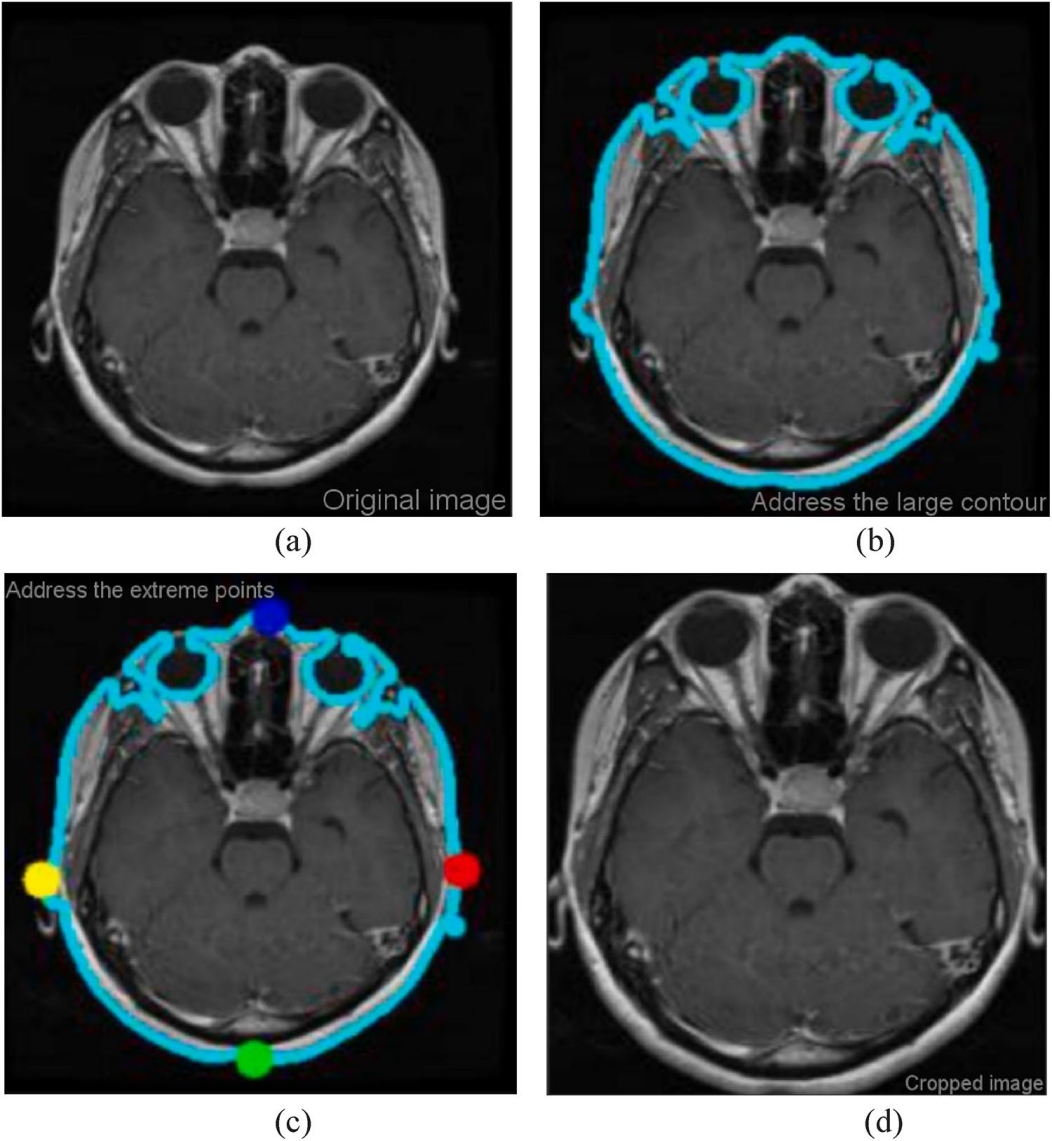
A series of steps is implemented to crop out unwanted regions MRI scans.
